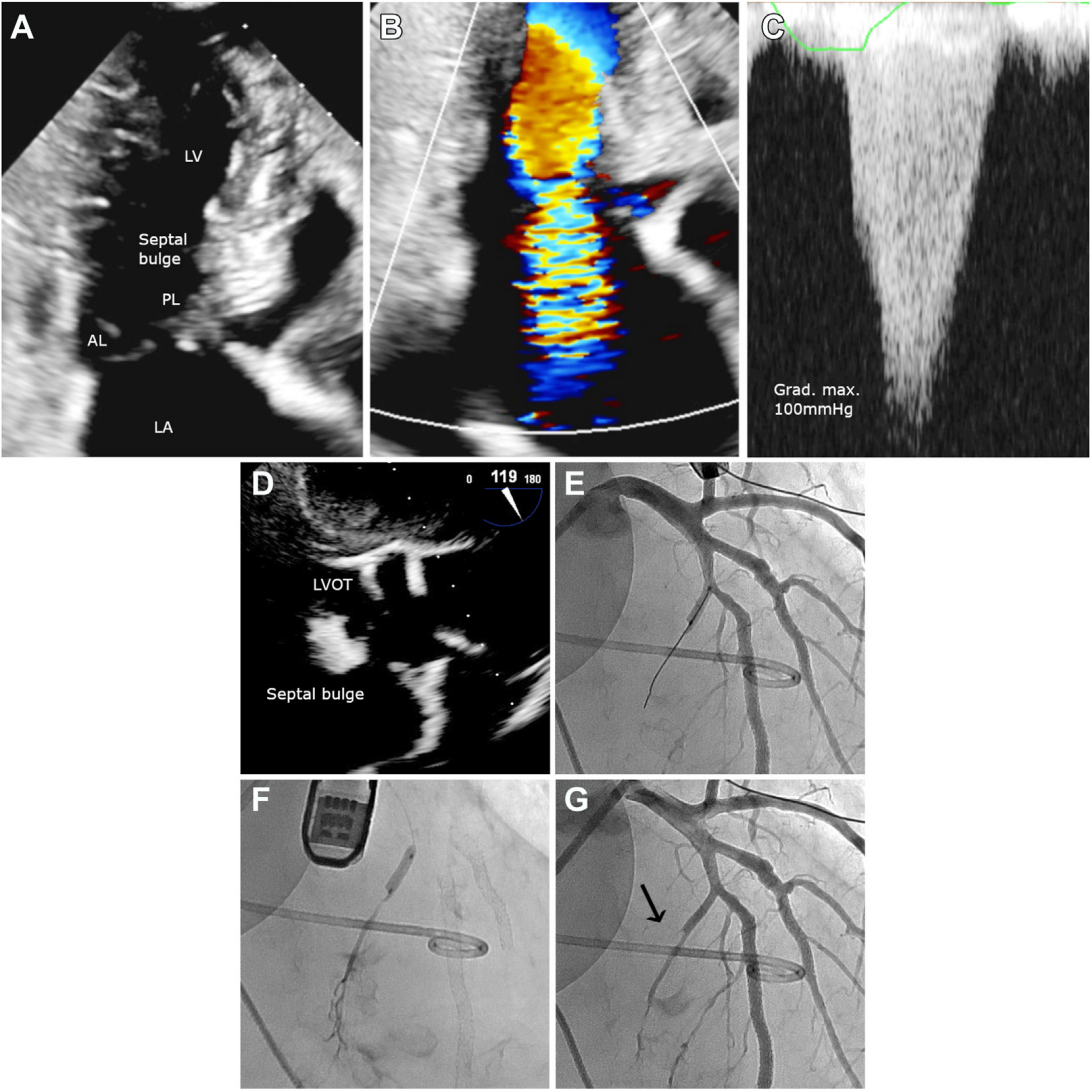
Transthoracic echocardiography after coronary revascularization and initiation of supportive treatment (top) and alcohol septal ablation under transesophageal echocardiographic guidance (bottom). (A) Systolic anterior motion phenomenon. (B) Severe mitral regurgitation. (C) "Dagger-shaped" envelope indicative of left ventricular outflow tract (LVOT) obstruction. (D) Transesophageal echocardiography with aortic valve long-axis view (119° probe) and contrast enhancement to localize the myocardium perfused by the septal artery. (E) Catheterization of the first septal perforator artery. (F) Ethanol injection in the first septal perforator artery. (G) Final result with no flow in the first septal perforator artery (arrow). AL, mitral anterior leaflet; Grad, gradient; LA, left atrium; LV, left ventricle; max, maximum; PL, mitral posterior leaflet.
